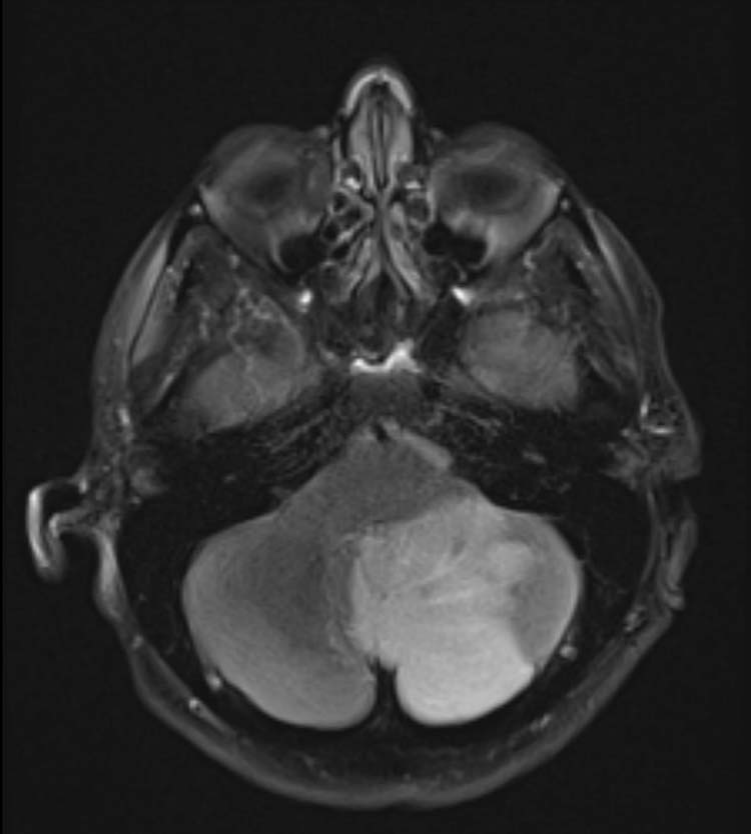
T2 sequence magnetic resonance imaging demonstrating large left cerebellar ischaemic stroke.
